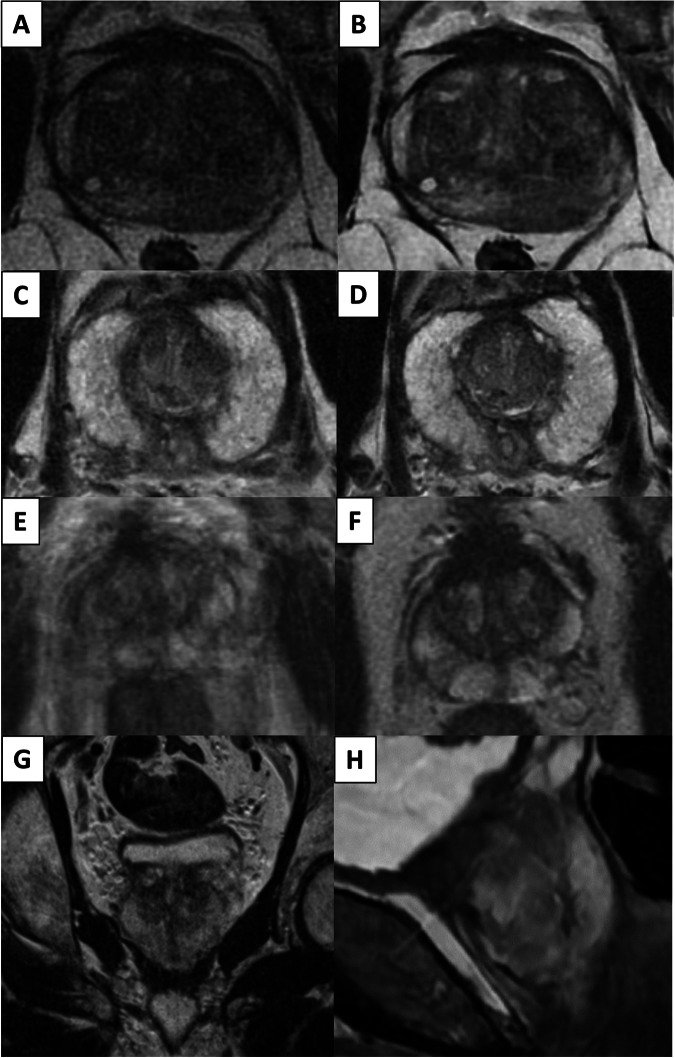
The four T2-WI criteria: examples with degraded axial (A, C, and E), coronal (G) and sagittal (H) T2-WI. Axial T2-WI of optimal image quality (B, D, and F). Axial T2-weighted images are from the same patients (before and after correction)Four criteria for DWI (maximum score: 4/4) (Fig. 3)
